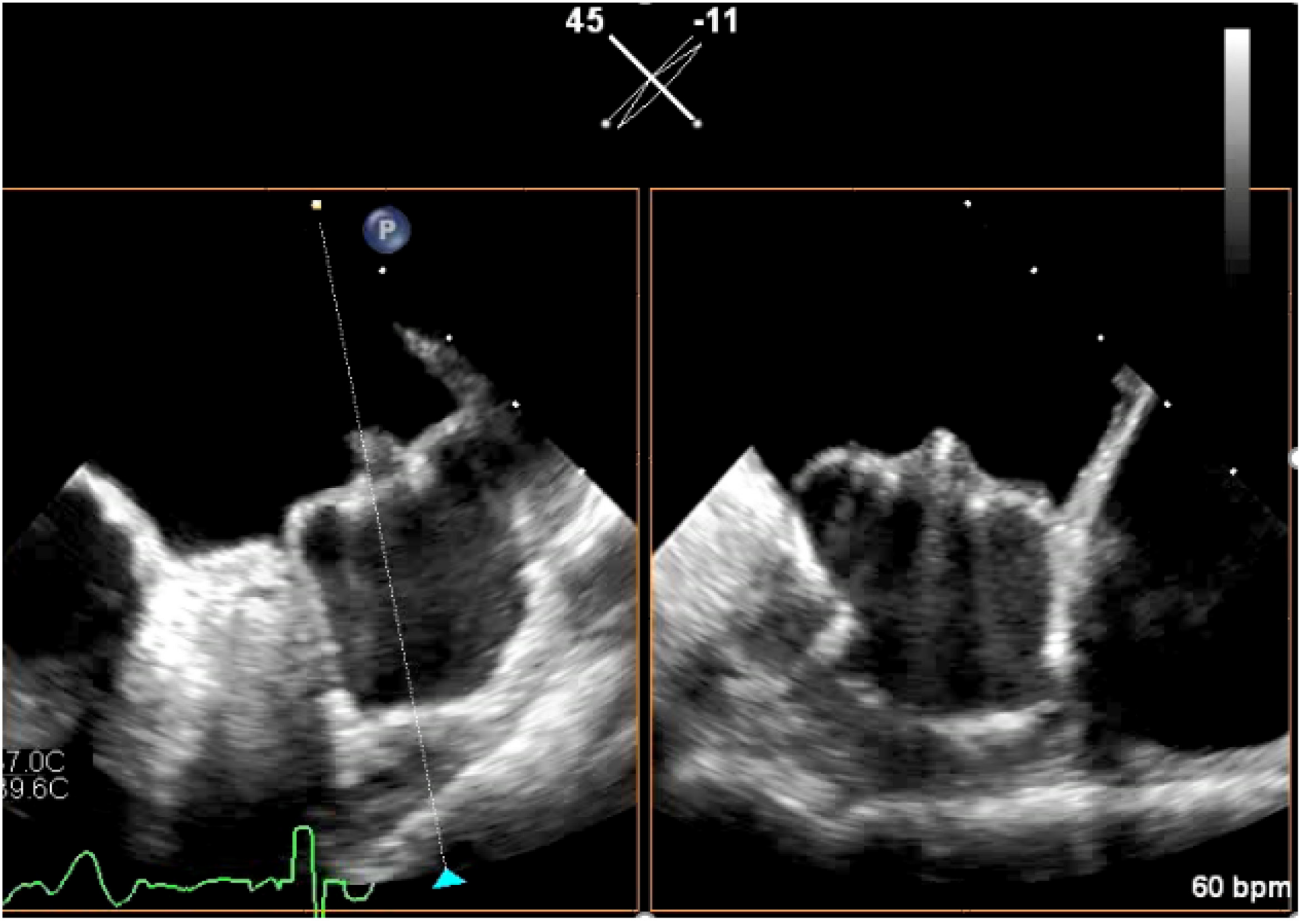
Transesophageal echocardiography image of the device after release. The device compression rate ranged 16.0%–22.9%, with no evidence of device leakage.
